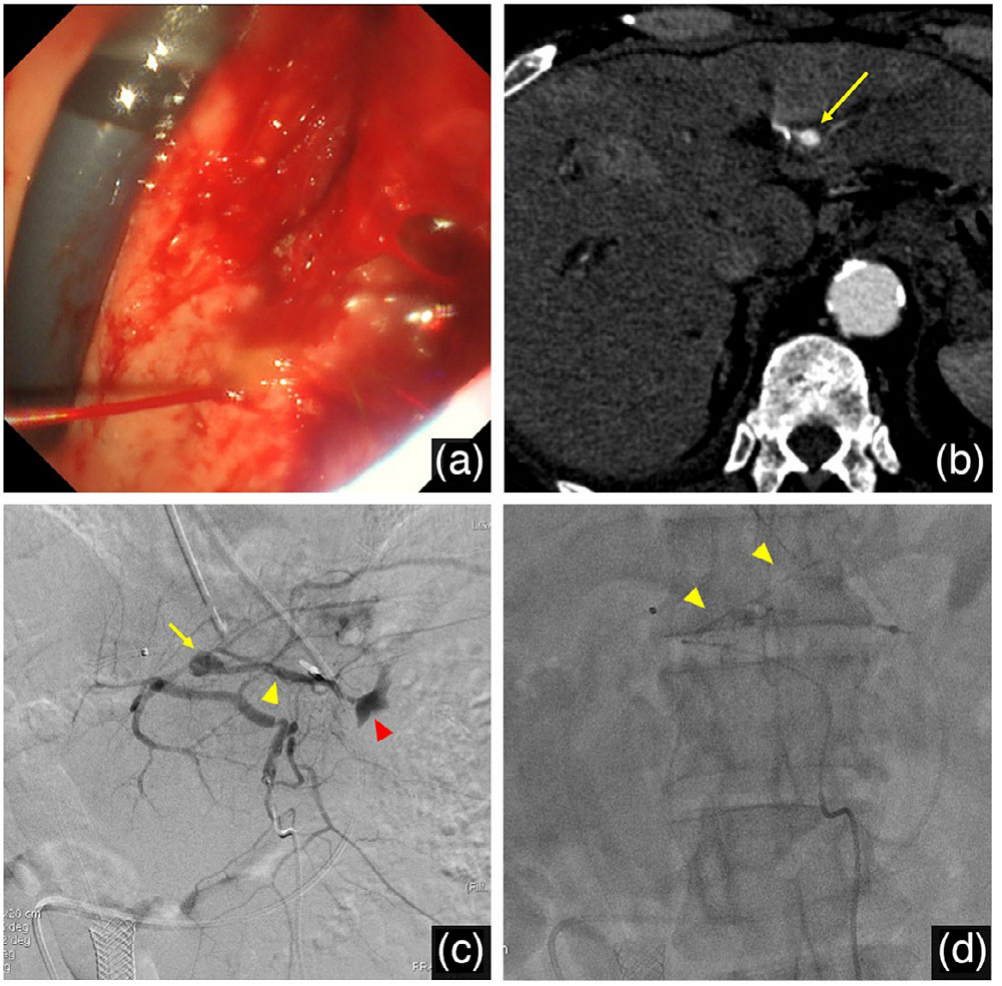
Bleeding complications and management. (A) Endoscopic view showing massive spouting bleeding from the endosonographically created route (ESCR) into the stomach. (B) Contrast‐enhanced computed tomography (CECT) scan revealing an 8 mm pseudoaneurysm (yellow arrow) in the A2 branch of the hepatic artery adjacent to the ESCR. (C) Angiography confirming a pseudoaneurysm (yellow arrow) with contrast extravasation from the ESCR (yellow arrowhead) flowing into the stomach (red arrowhead). (D) Post‐embolization angiography showing complete occlusion with *N*‐butyl‐2 cyanoacrylate (NBCA) cast formation (yellow arrowheads).
